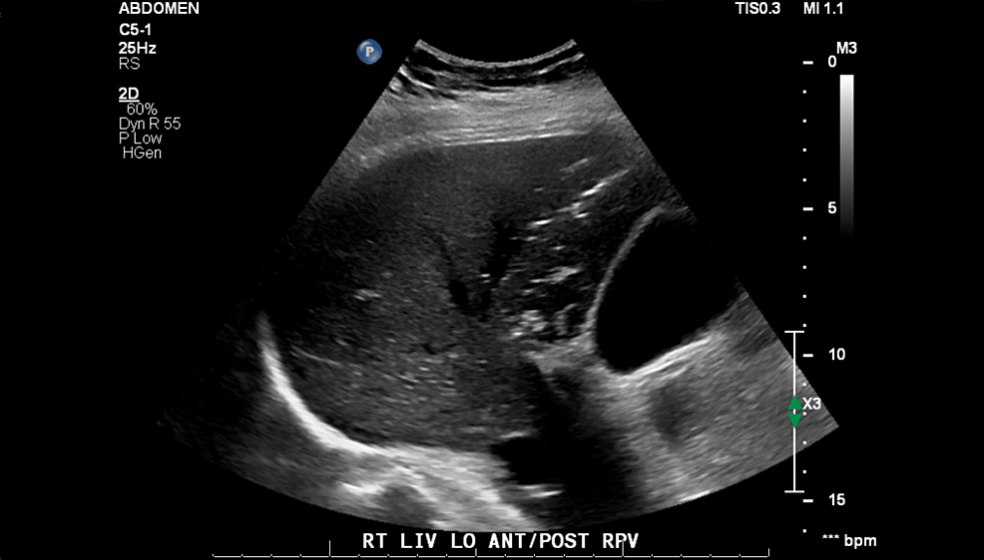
Liver ultrasound Liver ultrasound demonstrating air in the portal vein of the right lobe
